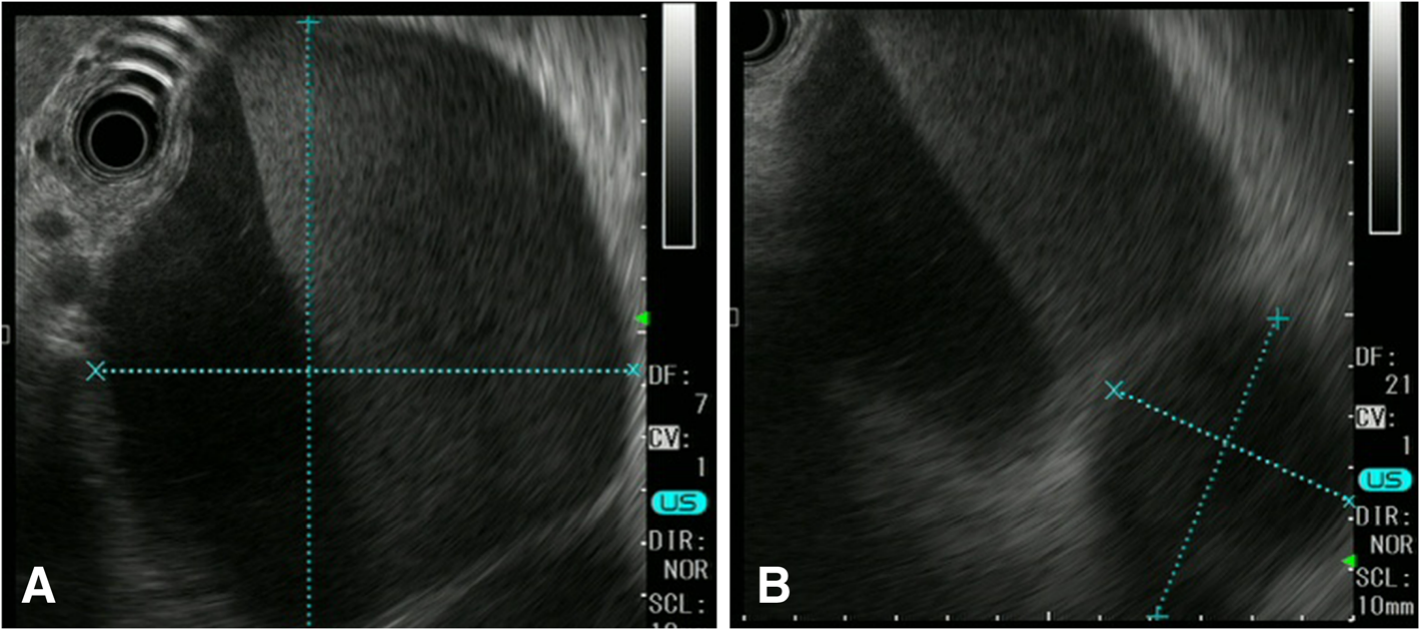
Endoscopic ultrasound (EUS) findings. a Pancreatic cyst. b Right renal cyst. EUS showed a liquid surface in the pancreatic cyst and did not show nodules in the cysts
